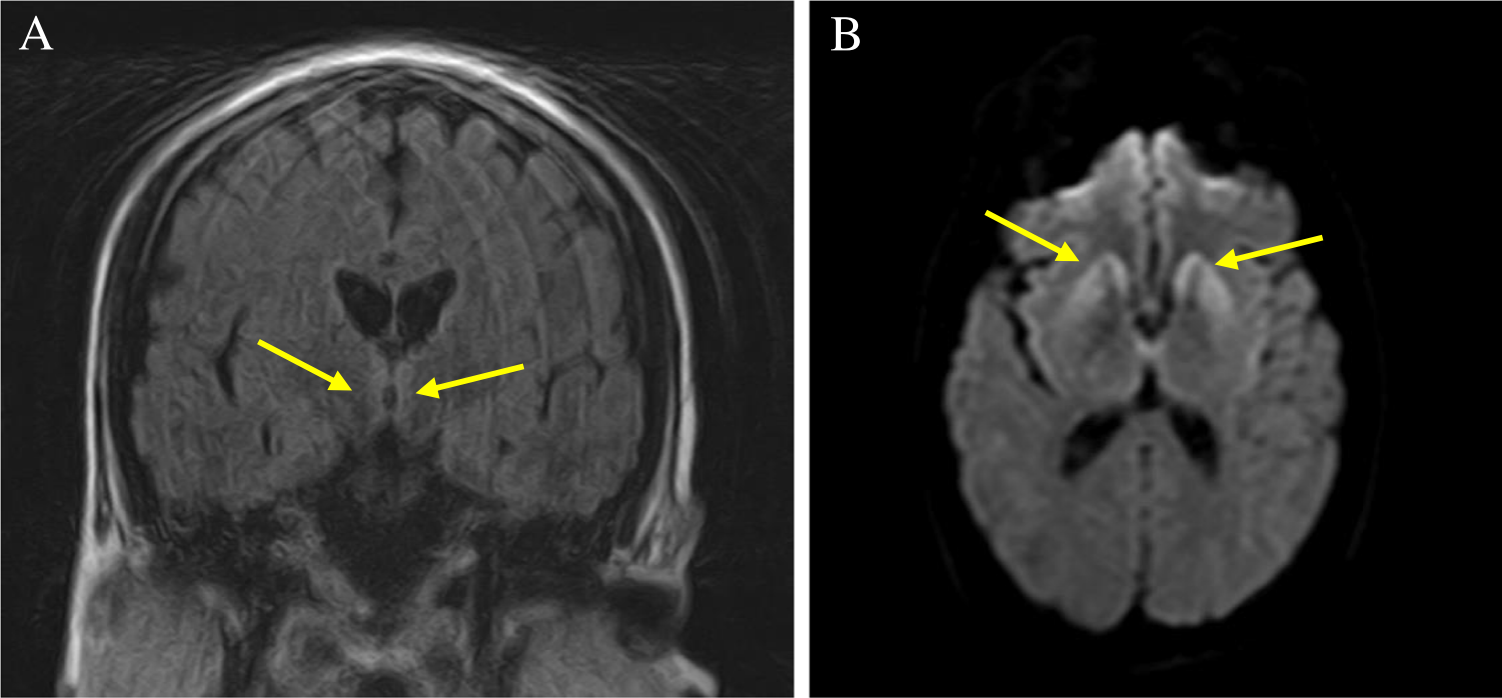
MRI brain of heterozygous (MV) variant CJD case. (A) Coronal FLAIR showing medial thalami hyperintensity only (arrows) and (B) Axial diffusion-weighted image demonstrating hyperintensity in the basal ganglia (arrows), medial thalami and bilateral insular cortices
